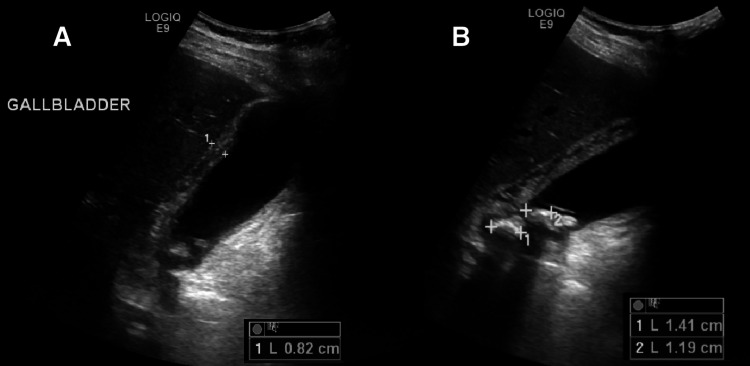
Ultrasound gallbladder showing acute calculous cholecystitis A: The gallbladder (GB) wall is thickened and edematous and measures 8.2 mm. Gallbladder is distended. B: Multiple stones are noted interspersed with sludge. The largest stone measures 14 mm. The rim of pericholecystic fluid is noted around the gallbladder.
